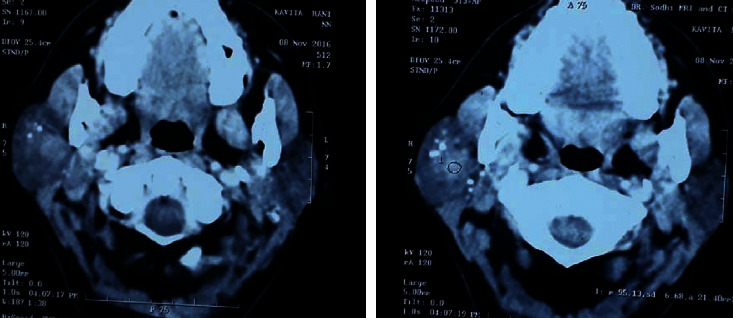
Axial cut contrast-enhanced computed tomogram showing a heterogeneously enhancing mass in the superficial lobe of the parotid gland with multiple pin head calcified foci in it.
